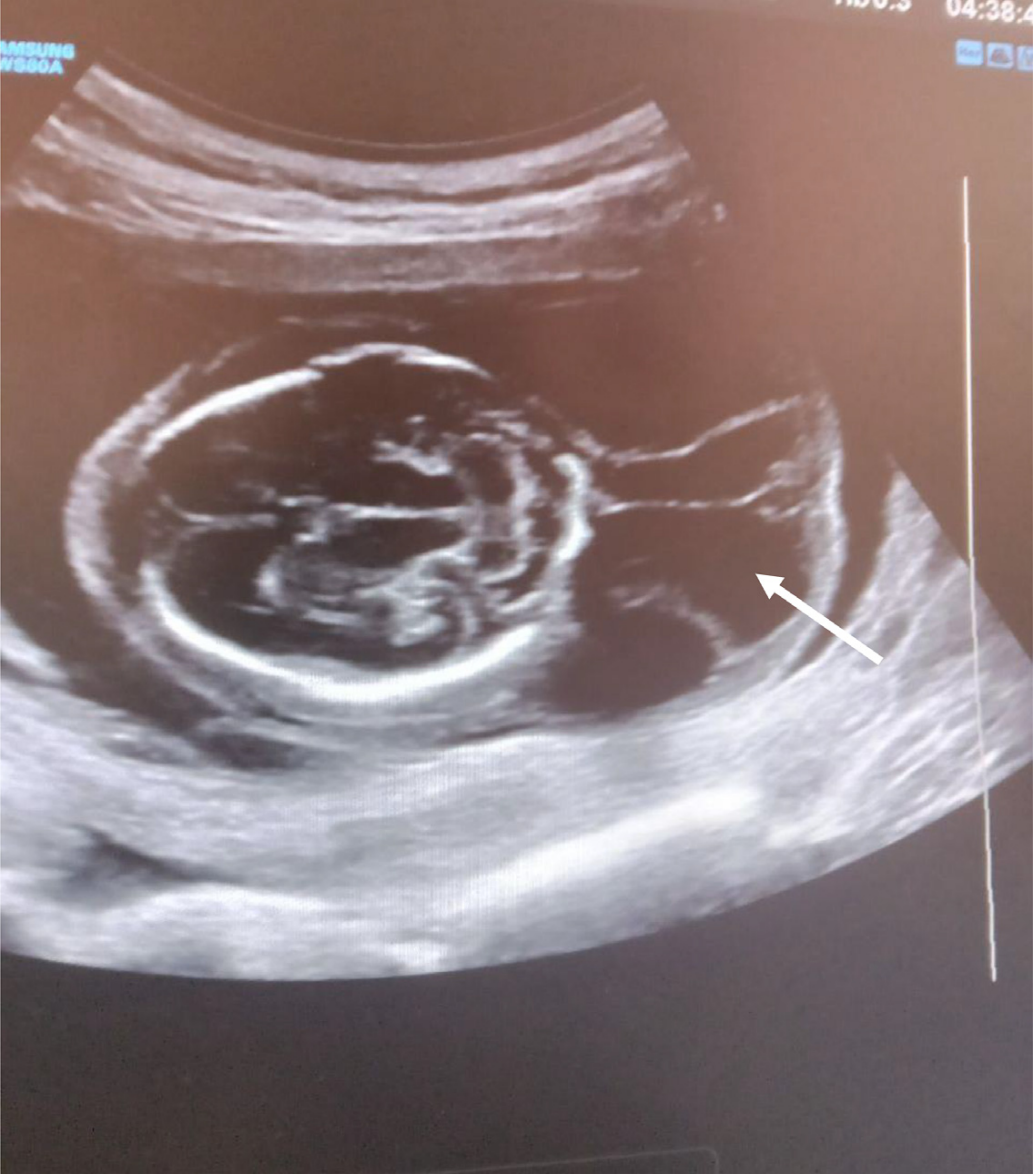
Multiseptated cervicoccipital cyst with no color Doppler flow (white arrow).
